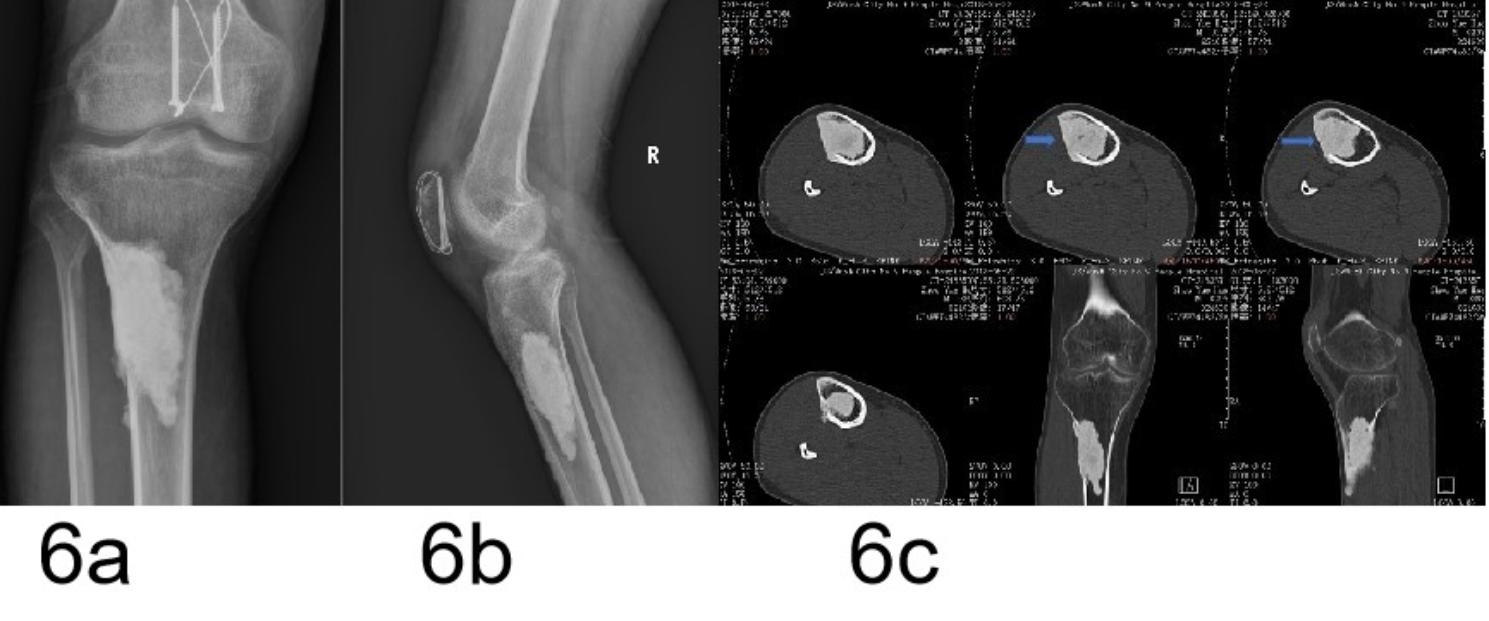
A 52-year-old male patient with a grade III IMSO. (A,b) AP and lateral radiographs showing cement spacer of PMMA inserted in the defects after resection for an upper tibial benign tumor. (c) CT scans showing continuous new bone formation (arrow) at nine years after insertion of the PMMA
